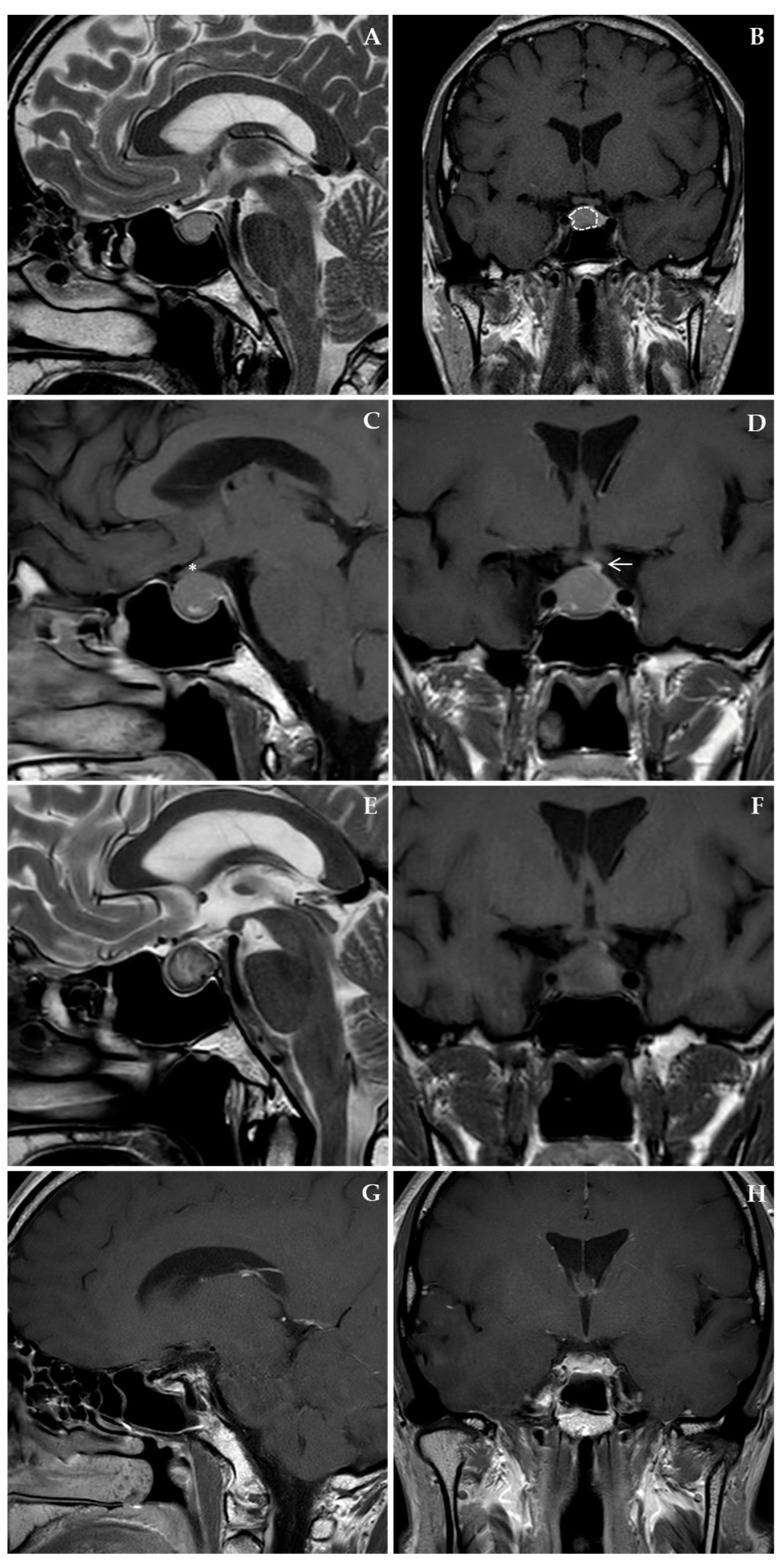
Brain MRI. (A) Sagittal T2-weighted images showing a macro-PitNET that is mildly hyperintense compared to the surrounding parenchyma. (B) Coronal T1-weighted contrast-enhanced images showing a macro-PitNET moderately hypointense compared to the surrounding parenchyma (dotted line). There is no evidence of residual healthy tissue between the lesion and the right cavernous sinus; the pituitary stalk appears deviated to the left, and the optic chiasm is within normal limits. (C) Sagittal and (D) coronal T1-weighted contrast-enhanced images showing an increase in the size of the macro-PitNET, with involvement of the right cavernous sinus, compression of the optic chiasm (asterisk), and persistence of pituitary stalk deviation (arrow). (E) Sagittal T2-weighted images and (F) coronal T1-weighted images contrast-enhanced, showing in the lateral left portion of the macro-PiNET a faint hyperintensity of the signal consistent with subacute bleeding. (G) Sagittal and (H) coronal T1-weighted contrast-enhanced images showing a marked reduction in the size of the adenohypophysis, with thin and peripheral enhancement post gadolinium.
